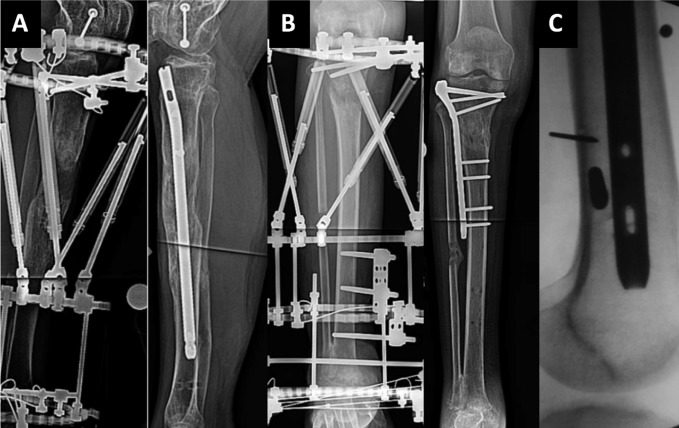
Radiographs demonstrating (A) lengthening and then nailing and (B) lengthening and then plating techniques. C, Fluoroscopic image demonstrating lengthening over nail with external pins avoiding the intramedullary nail.
